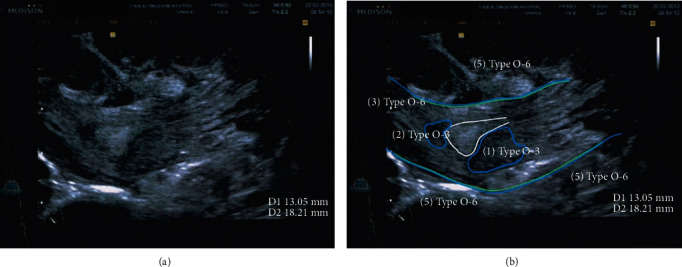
(a) Abdominal ultrasound (sagittal view of right lower abdomen) revealed an 18-millimeter-thick inhomogeneous endometrium with multiple intramural and subserosal leiomyomata (2 weeks after the combined regimen for medical abortion was administered). (b) Ultrasonic imaging revealing multiple leiomyomata (white line: endometrium; blue line: leiomyomata; green line: uterine wall) (Samsung Medison).
